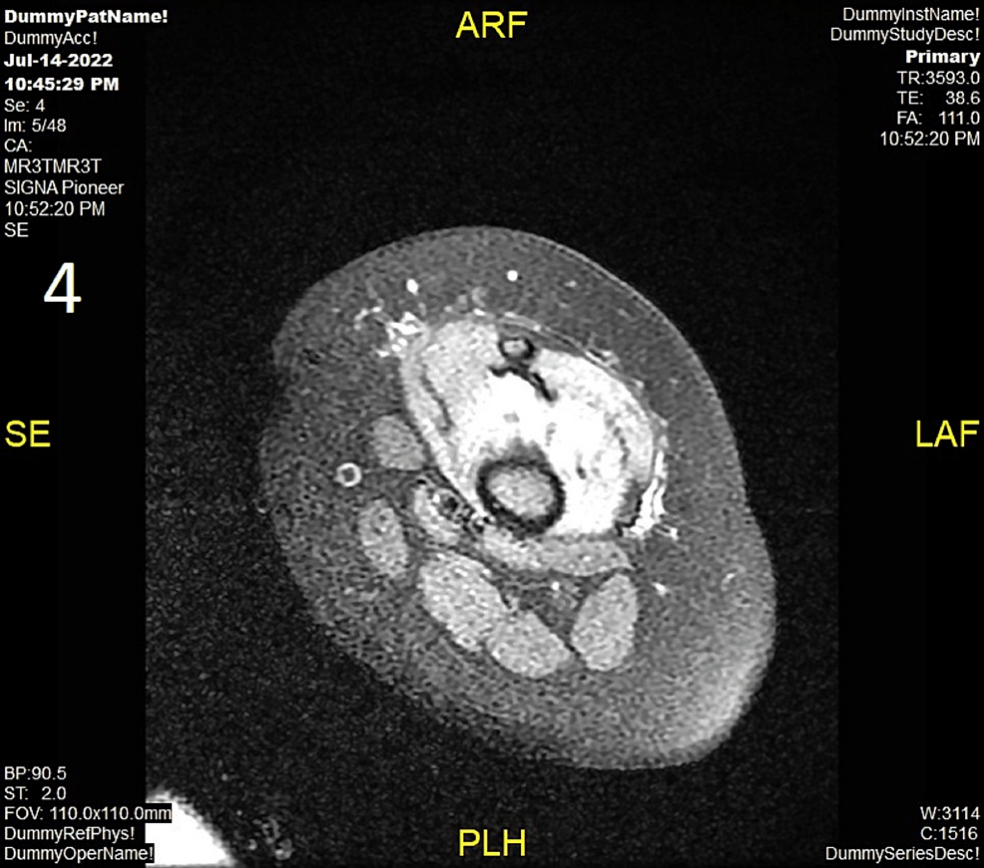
MRI of the right knee joint There is synovial thickening, enhancement, and moderate joint effusion with surrounding soft tissue edema. The visualized bones show no significant bone marrow edema, periosteal new bone formation, or abnormal enhancement.
